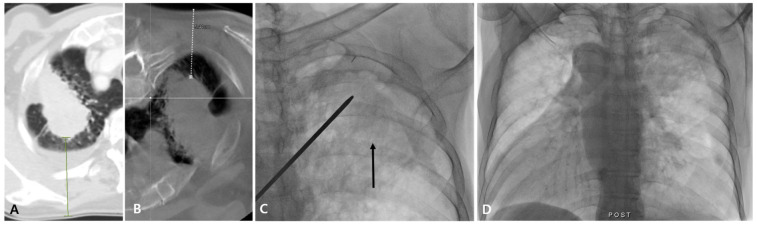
A >10 mm difference in the lung nodule lesion depth was observed via fluoroscopy-guided PTNB with the aid of planning CBCT in a 75-year-old man with lung adenocarcinoma. A diagnostic CT image (A) shows a 69 mm lesion depth (green line), and a planning CBCT image in the prone position with scapular lateral rearrangement (B) shows a 54 mm lesion depth (white dashed line) for a 70 mm mass in the left apex. On fluoroscopy, the mass is identifiable (arrow) (C), and PTNB was performed based on the 54 mm lesion depth observed via CBCT. After PTNB, no immediate PTNB-related complications occurred (D), and diagnostic success was achieved.
